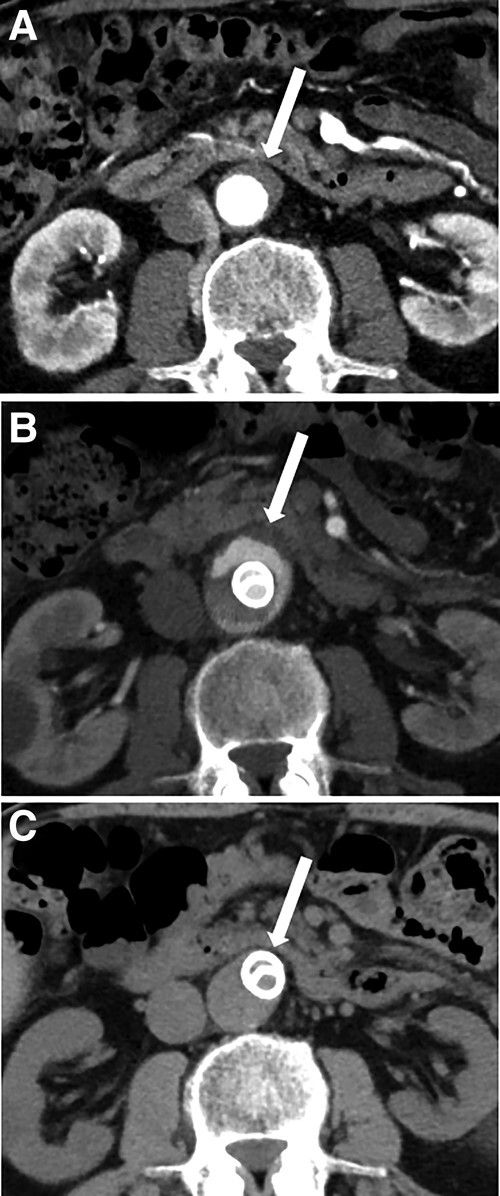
Abdominal aneurysmal regression in follow-up CT images. (*A*) The true lumen of infrarenal aorta was totally collapsed before the procedures (arrow). (*B*) CT angiography demonstrated aneurysmal formation and type Ⅲ endoleak in the true lumen after the TEVAR procedure. (*C*) True lumen aneurysm regressed at 1-year follow-up.
